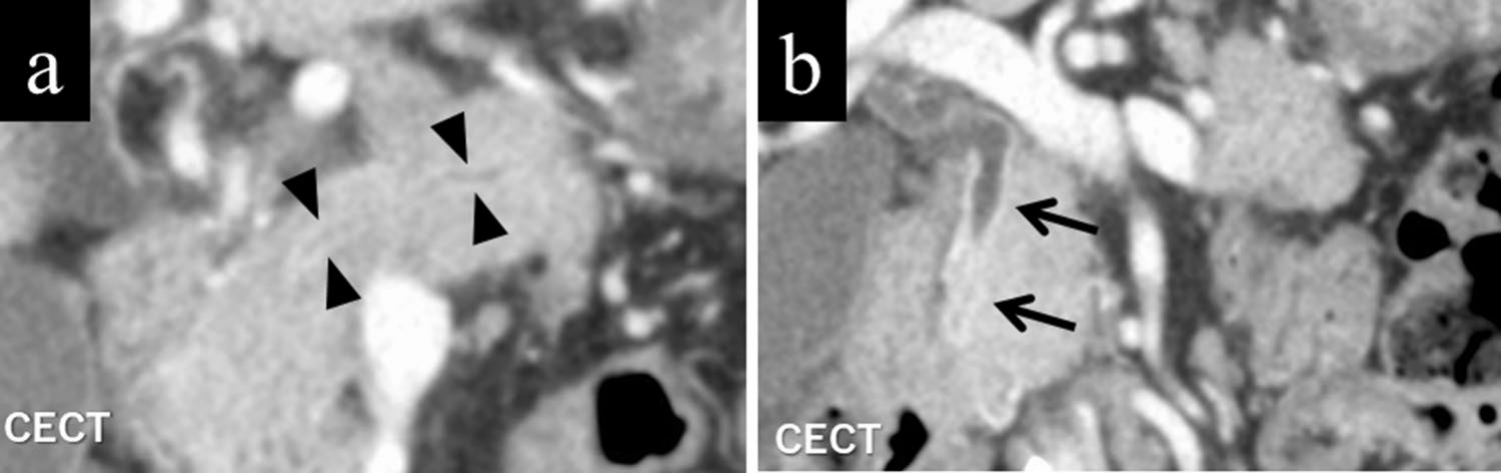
Coronal reconstruction images of contrast-enhanced portal-venous phase CT of a male in his 70 s with typical autoimmune pancreatitis. a Contrast-enhanced segments demarcating the wall of the main pancreatic duct (enhanced duct sign) are observed (arrowhead). b The enhancement demarcating the common bile duct wall is also evident (arrows), reflecting coexisting sclerosing cholangitis
